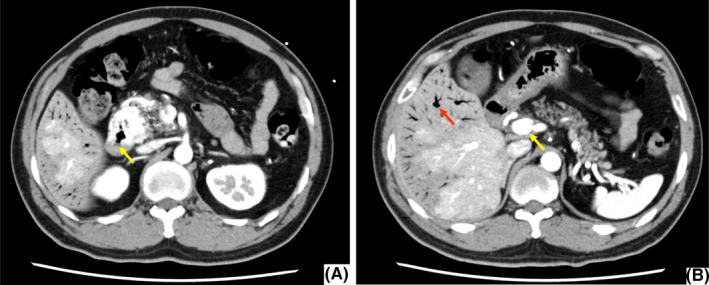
Arterial‐phase images of DCE‐CT. A, A hypervascular lesion in the head of the pancreas extending into the duodenal ulcer (yellow arrow). B, Early enhancement of the portal vein (yellow arrow) and portal venous gas in the liver (red arrows). Swelling of pancreas is not present
